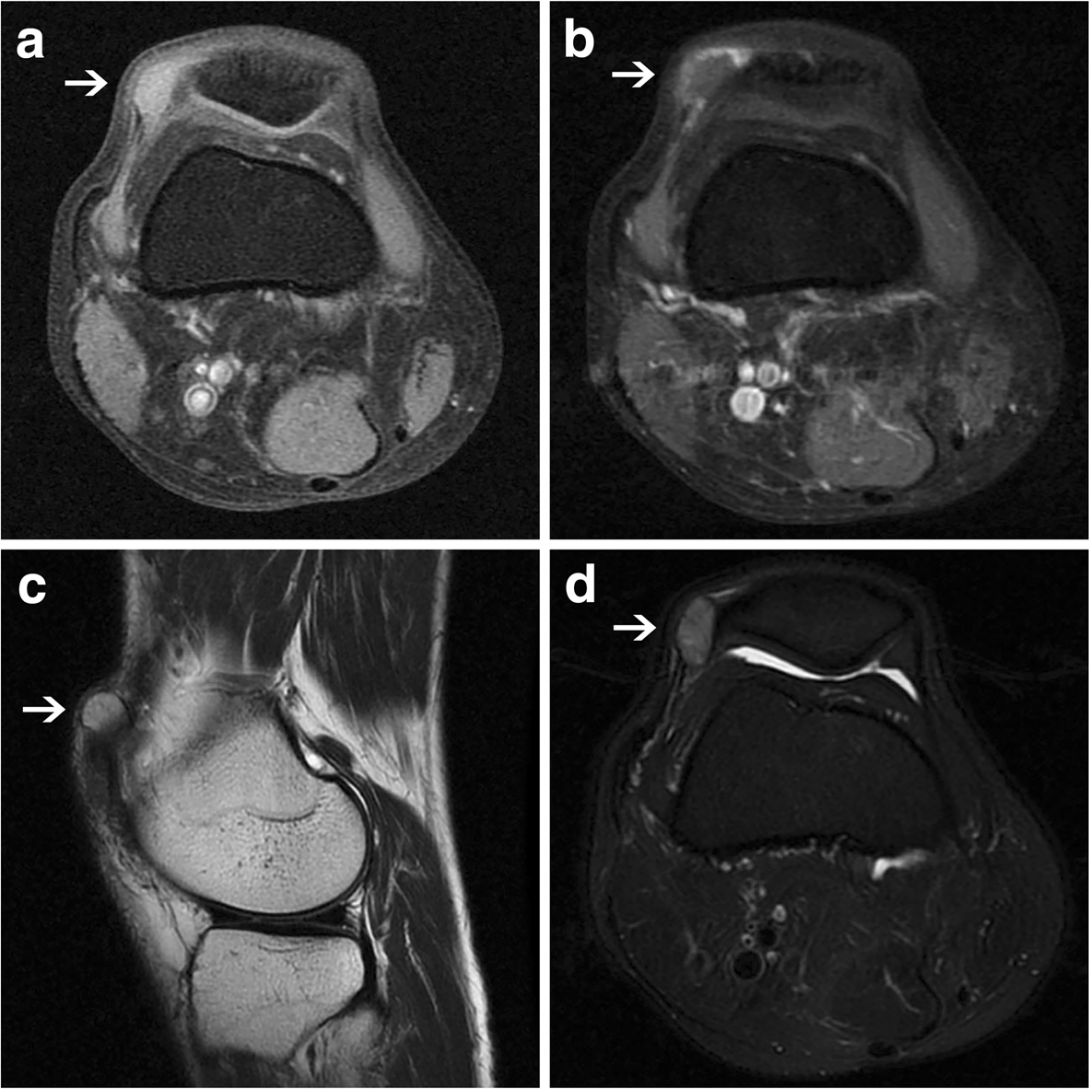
MRI showed the mass presented isointense signal to muscle on STIR T1 sequence (a), and was heterogeneously distinctly strengthened on enhanced STIR T1 sequence (b). The MRI also showed slightly hyperintense signal on T2WI (c) and STIR T2 sequence (d)
